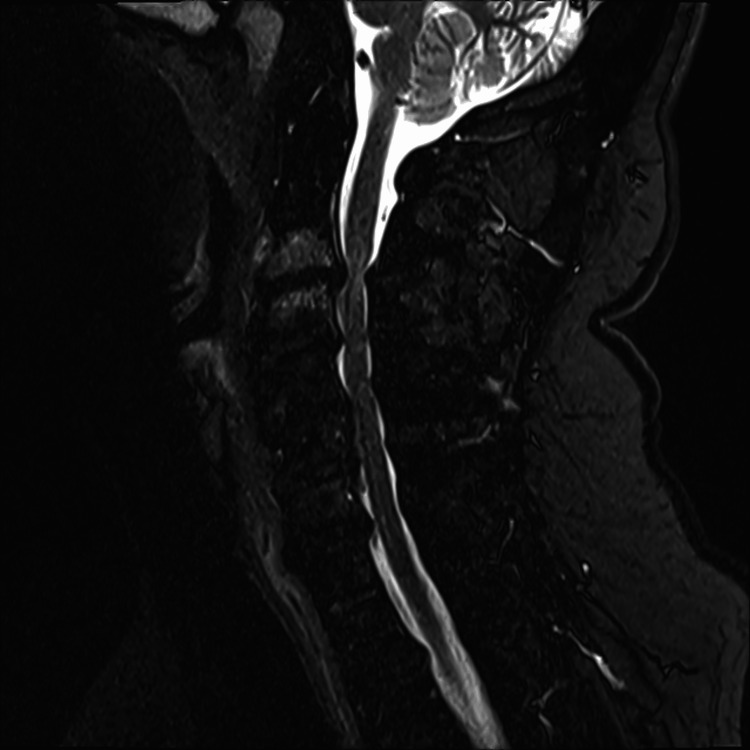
Sagittal short tau inversion recovery (STIR) MRI of the cervical spine.
